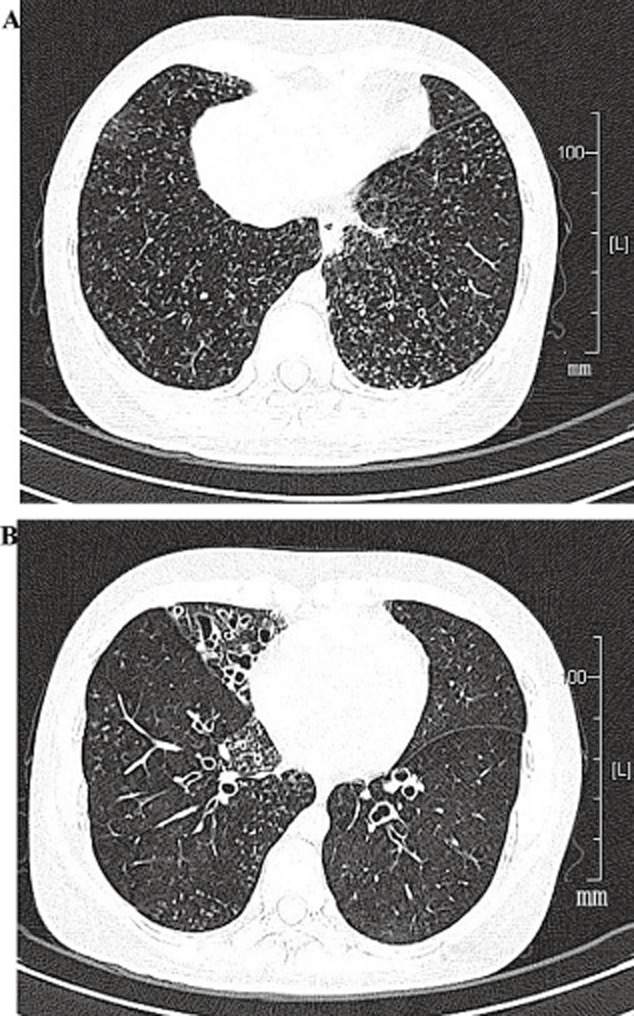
Computed tomography (CT) images of the chest. (A) High-resolution computed tomography (HRCT) shows bronchiectasis and bronchiolitis, ring-shaped or ductal opacities in upper, middle and lower lungs, some of which are accompanied by small nodules. (B) Bronchiectasis involved in right middle lobe.
